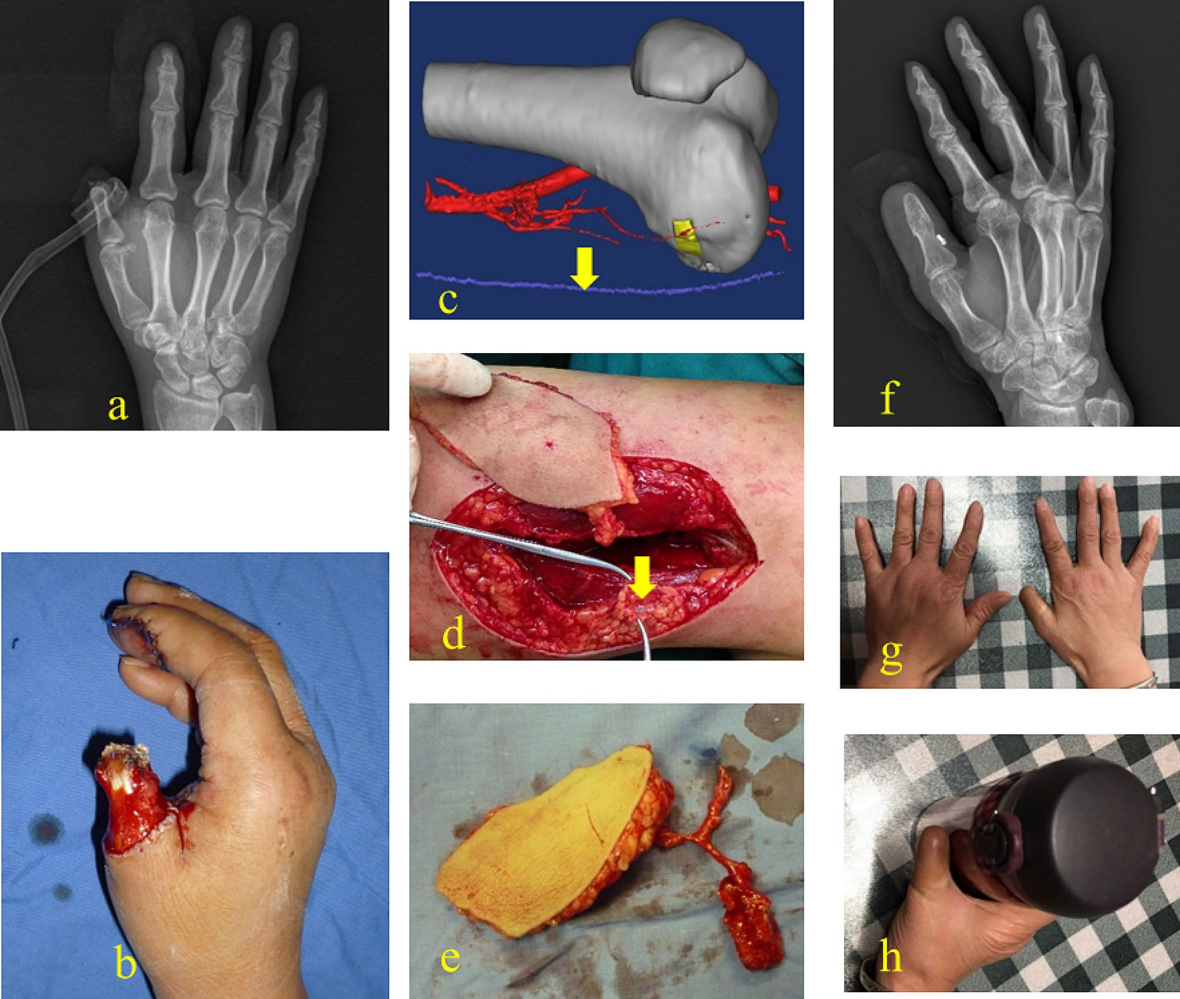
Case 1. Bone and skin defect of the right thumb before reconstruction (a, b). Preoperative plan of the chimeric DGAP flap using Mimics 20.0 with CTA data. The saphenous vein (yellow arrow) (c). Intraoperative photograph shows that the saphenous vein (yellow arrow) was preserved in the donor site (d), and the chimeric DGAP flap was harvested (e). Postoperative CTA of the recipient area, SB, skin artery branch, and OB (f). Radiograph of the same hand showing bony union 72 months after reconstruction and screw removal (g). Final outcome of the same thumb after reconstruction with a satisfactory appearance (h)
